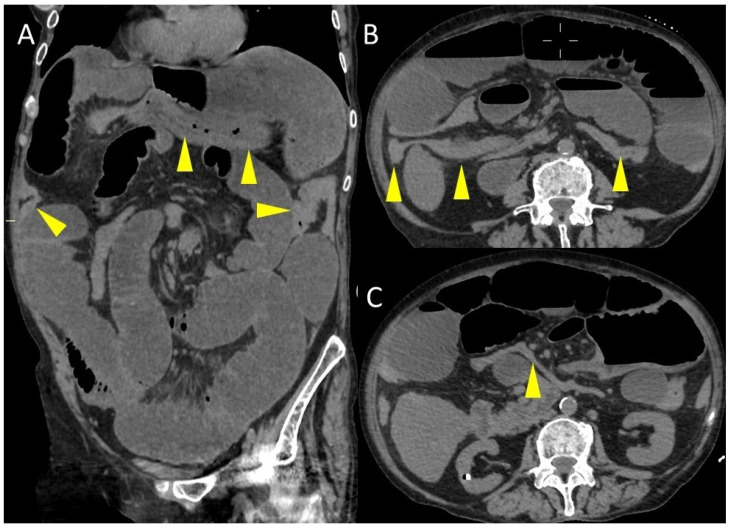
Coronal (A) and axial (B,C) CT images. The yellow arrowheads indicate the colon, which is collapsed throughout its course.
